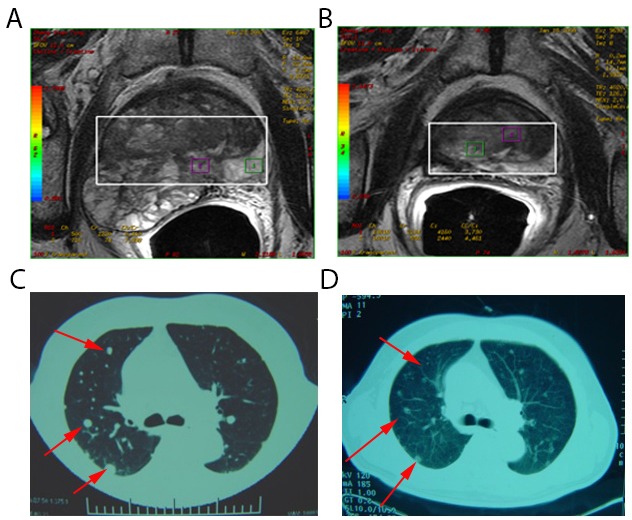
Spontaneous regression of lung metastases after cryoablation for metastatic prostate cancer. (A) Pelvic magnetic resonance imaging of a 78-year-old man with metastatic prostate carcinoma (Stage T3bN0M1c). This lesion was treated with cryoablation. (B) Pelvic magnetic resonance imaging 1 month post-treatment appeared inactive. (C) Computed tomography scans of pulmonary metastasis of the patient before treatment revealed multiple metastases in lung tissues (red arrow). (D) Computed tomography scans of pulmonary metastasis of the patient 1 month post-treatment indicated regression of lesions in lung tissues (red arrow).
